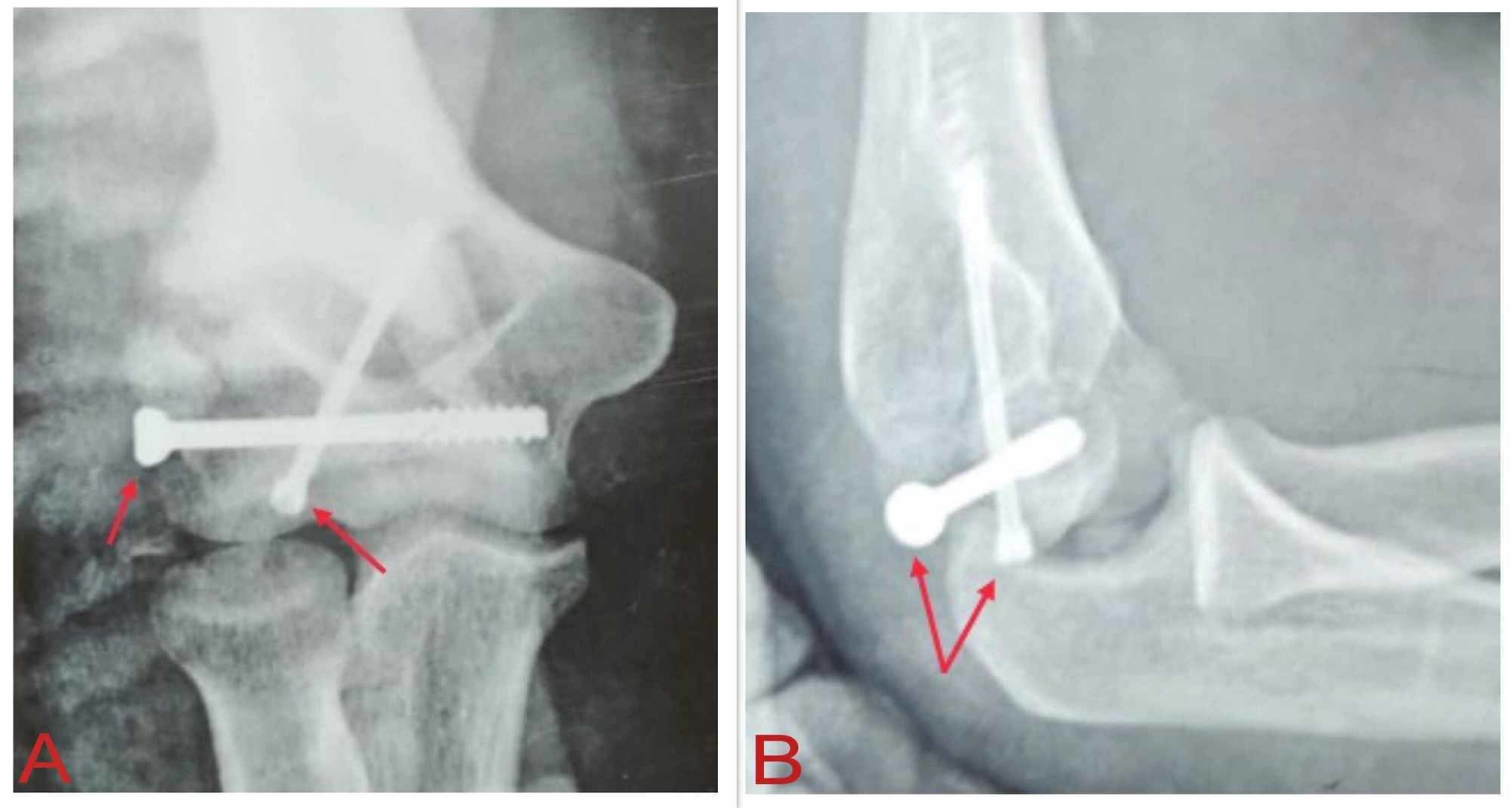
X-rays after open reduction and screw fixation of the capitellar fracture. The arrows denote the screws used for fixation in the (A) anteroposterior and (B) lateral views.
